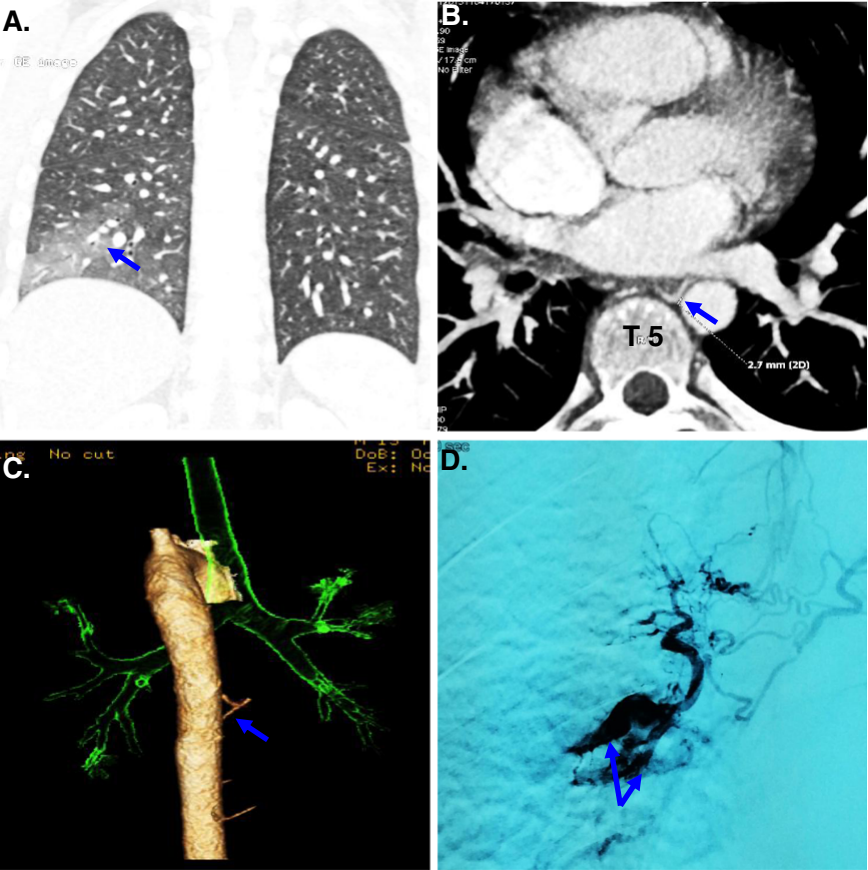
Radiolographic findings of Dieulafoy’s disease of the bronchus. (A) A chest CT showed exudative lesion, consolidation and atelectasis of the right lower lobe; (B, C) A CT angiography of bronchial artery showed a right bronchial artery arising from the anterior wall of the thoracic aorta at T5 level; (D) Angiography of the bronchial artery showed that a distal right branch of the bronchial artery arising from the thoracic aorta at T5 level was dilated and tortuous.
